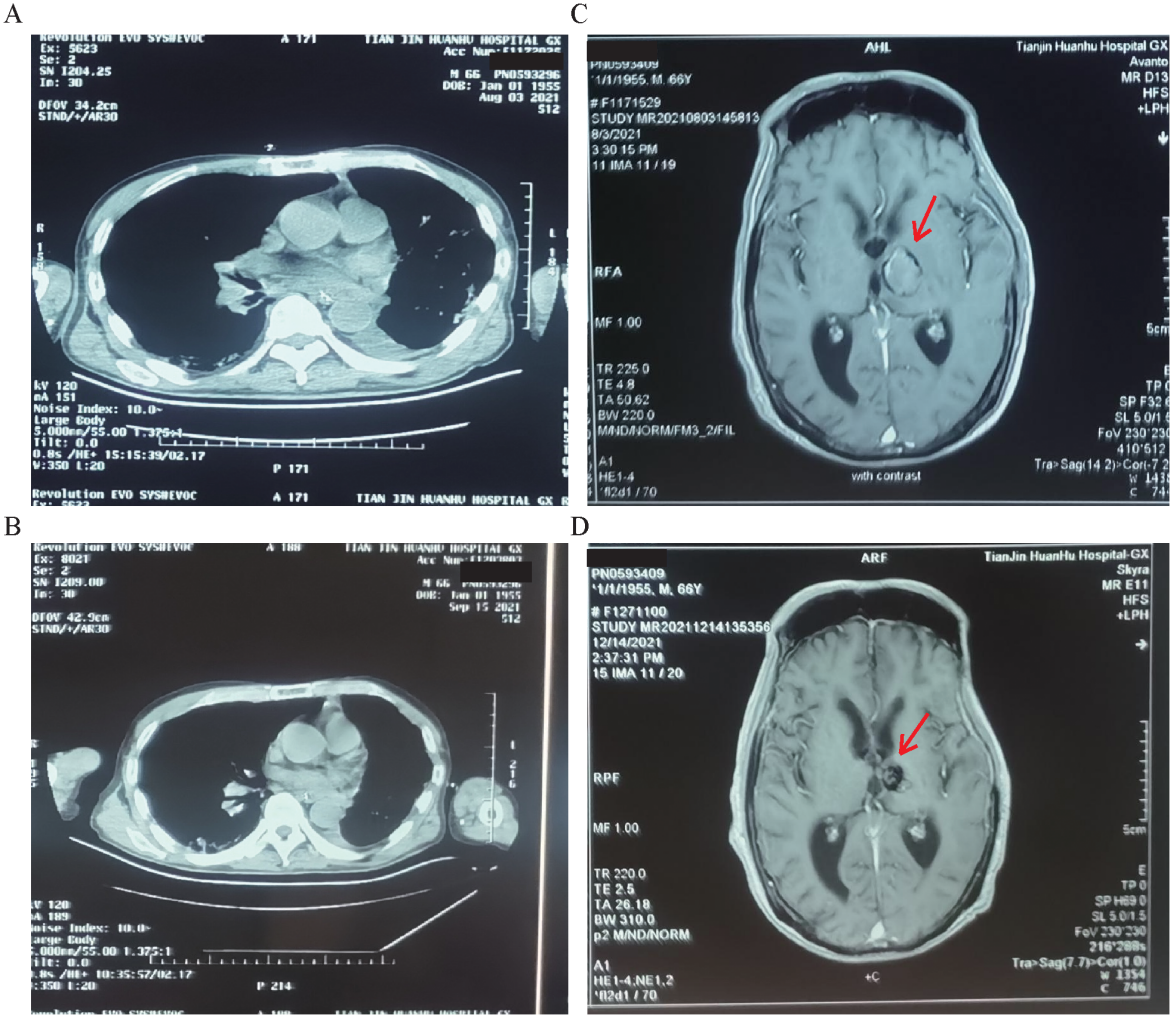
Chest CT and head MRI results of case 11. (A, B) Chest CT and MRI results before immunotherapy combined with anti-angiogenic therapy. (C, D) Chest CT and MRI results after four cycles of immunotherapy combined with anti-angiogenic therapy. Arrows point to the location of the lesion.
